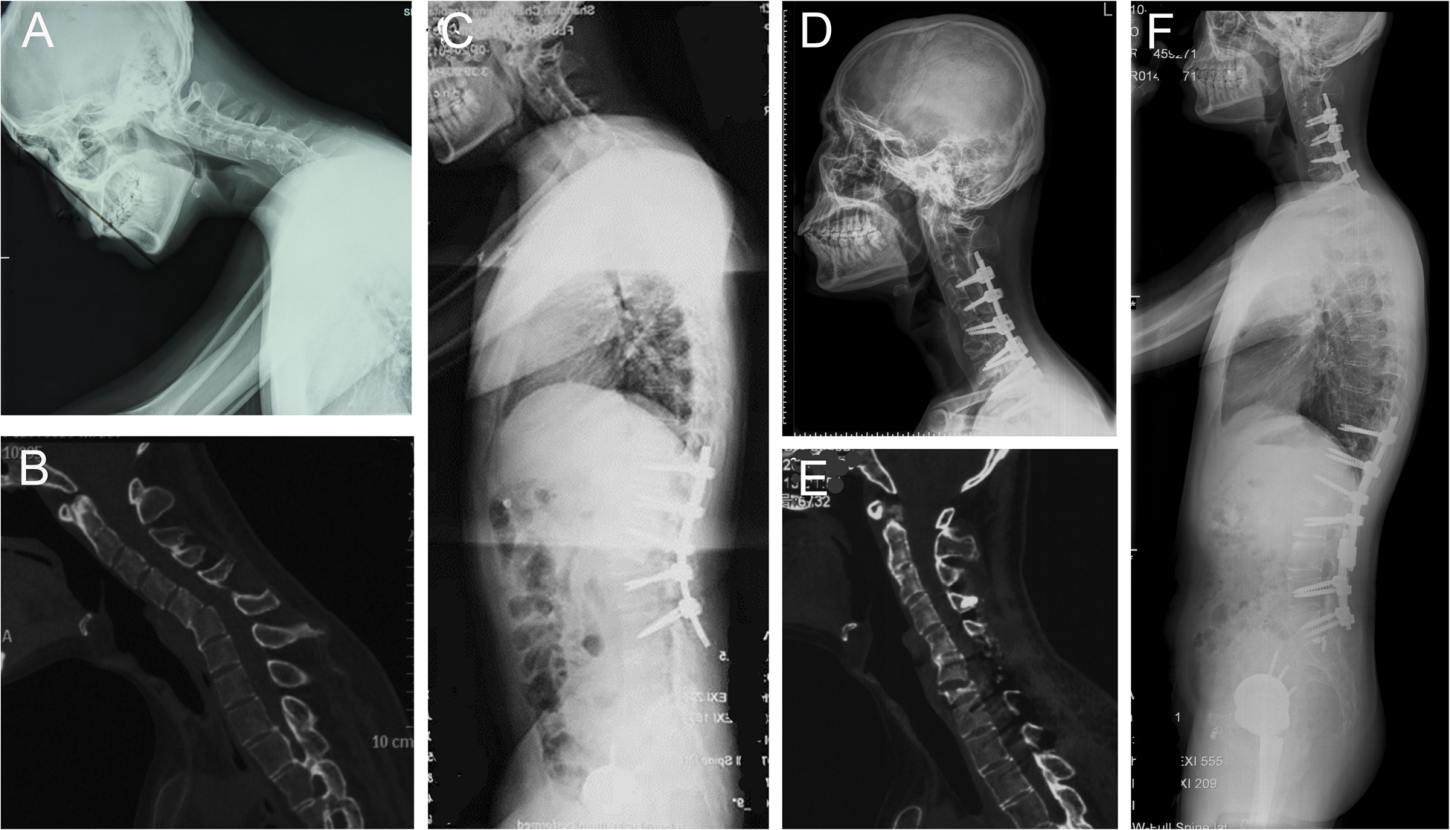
Pre- and post-operative radiological outcomes and clinical photographs. a-c A patient with cervicothoracic kyphosis secondary to ankylosing spondylitis presented with restriction of forward gaze. d-f Modified PSO was performed at C7, and radiographs at 2 years follow-up revealed significant improvement of the deformity
